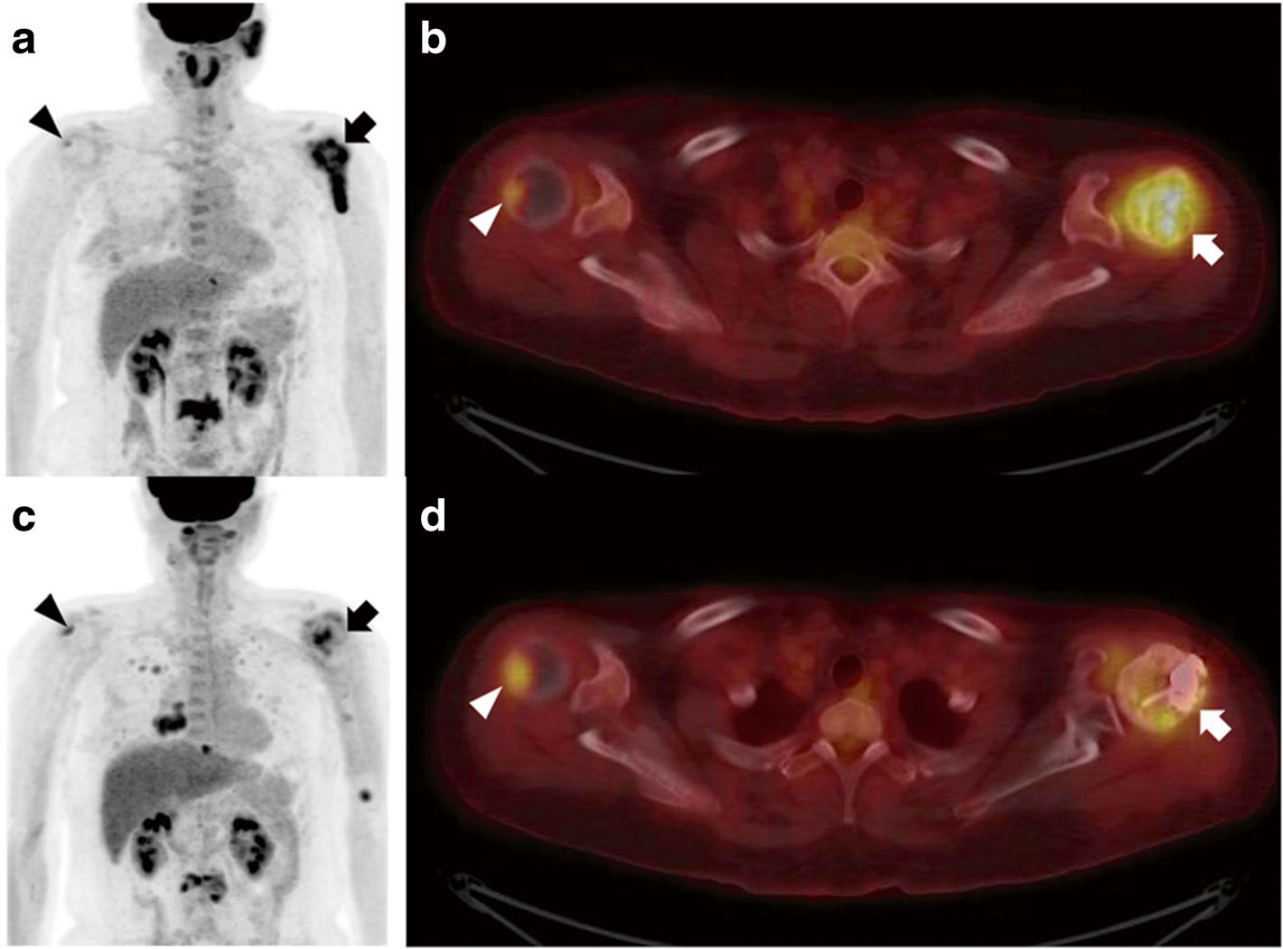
Suppression of tumor progression by self-locking screws with PC. A 48-year-old woman with multiple bone metastases due to salivary gland tumor. Preoperative maximal intensity projection (MIP) (a) and transaxial (b) images of F-18-FDG PET/CT show hypermetabolic lesions in the left (arrow; maximum standardized uptake value [SUVmax] = 11.4) and right (arrowhead; SUVmax = 2.8) proximal humerus. Six months postoperatively, the MIP (c) and transaxial (d) images of F-18-FDG PET/CT demonstrated tumor suppression of the right proximal humus, the op. site (arrow; SUVmax = 5.5). However, naive metastatic area left proximal humerus (arrowheads; SUVmax = 3.8) reveal tumor aggravation of bone metastasis, and multiple new metastatic lesions were found in the lungs and T10 and L3 spines
